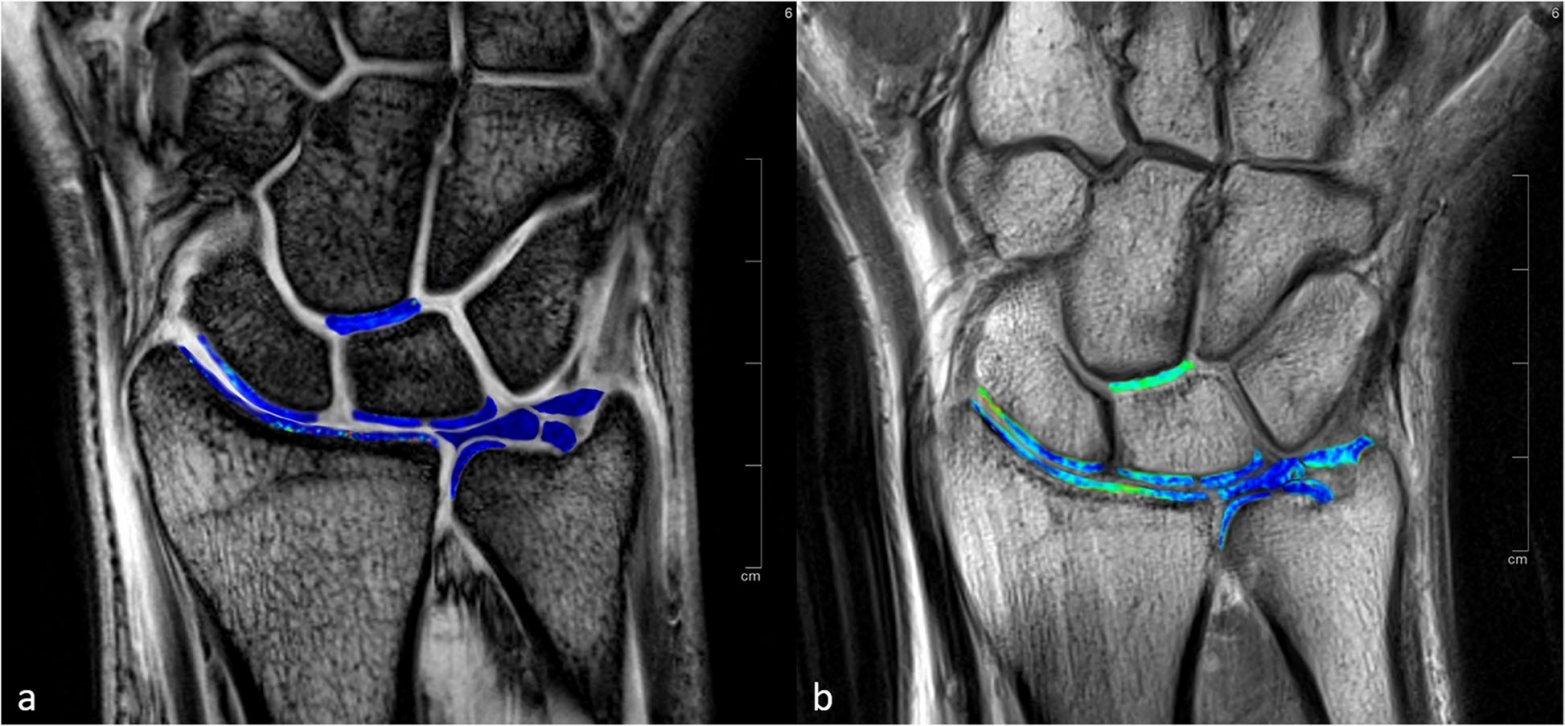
Three-Tesla images of the first echo of the T2*-weighted multi-echo gradient-echo sequence (a) and T2-weighted multi-echo spin-echo sequence (b) of a healthy volunteer with superimposed color-coded maps of T2* and T2 values. Regions of interest (ROIs) were placed in the cartilage of the distal radius, in the proximal scaphoid, in the proximal radial and ulnar lunate, in the distal radioulnar joint, and in between the distal lunate and proximal capitate. Three ROIs were placed at the triangular fibrocartilage complex: at the central disk and at both the foveal and apical attachments. The same analysis was performed for 7-T MRI
